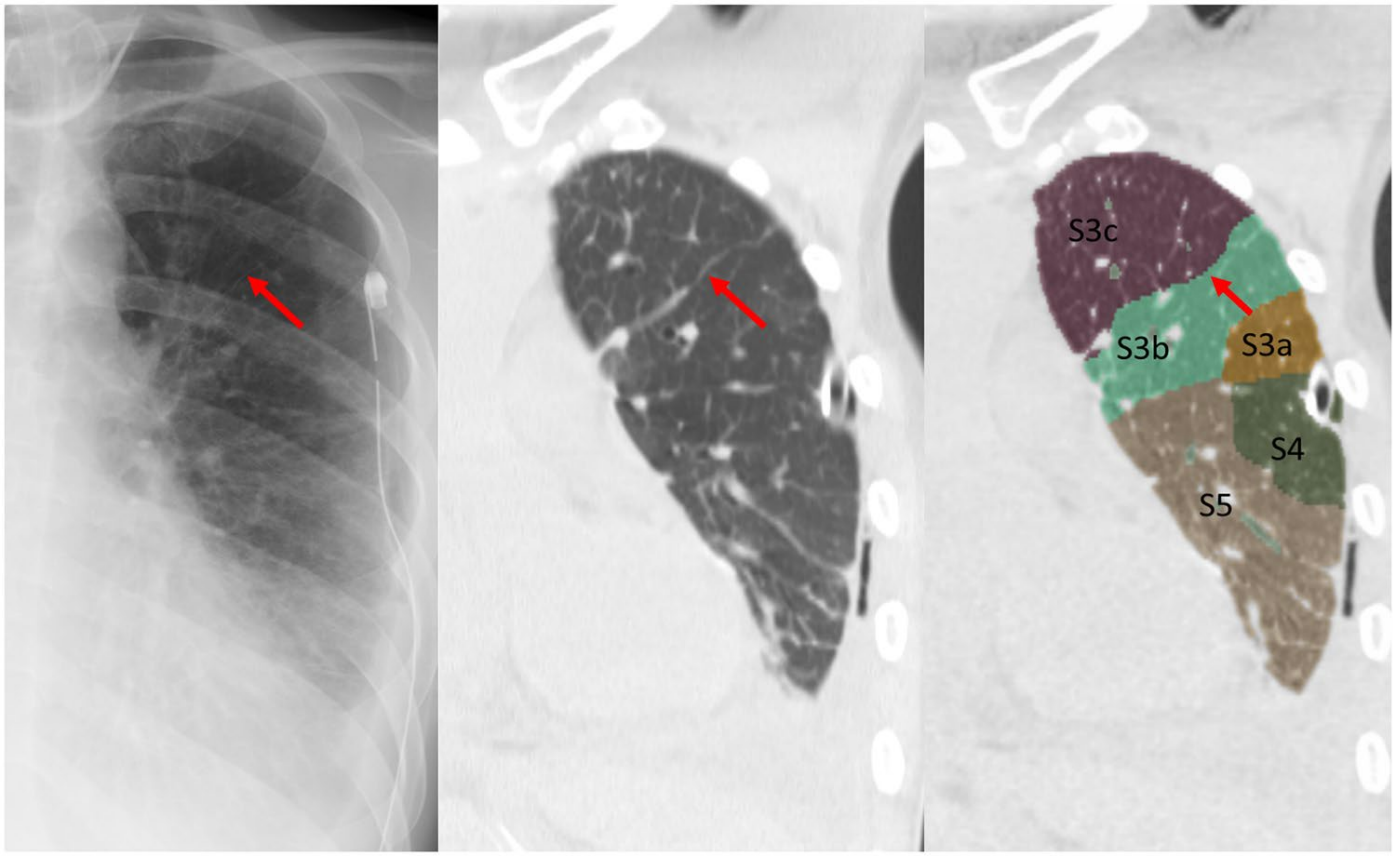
Case 5. Line 8. 35-year-old man with tongue cancer. Chest X-ray shows the Kerley A-line in the left lung. The line corresponds to the thickened septal line on CT and matched with the segmented lines between S3b and S3c by 3D segmentation analysis
